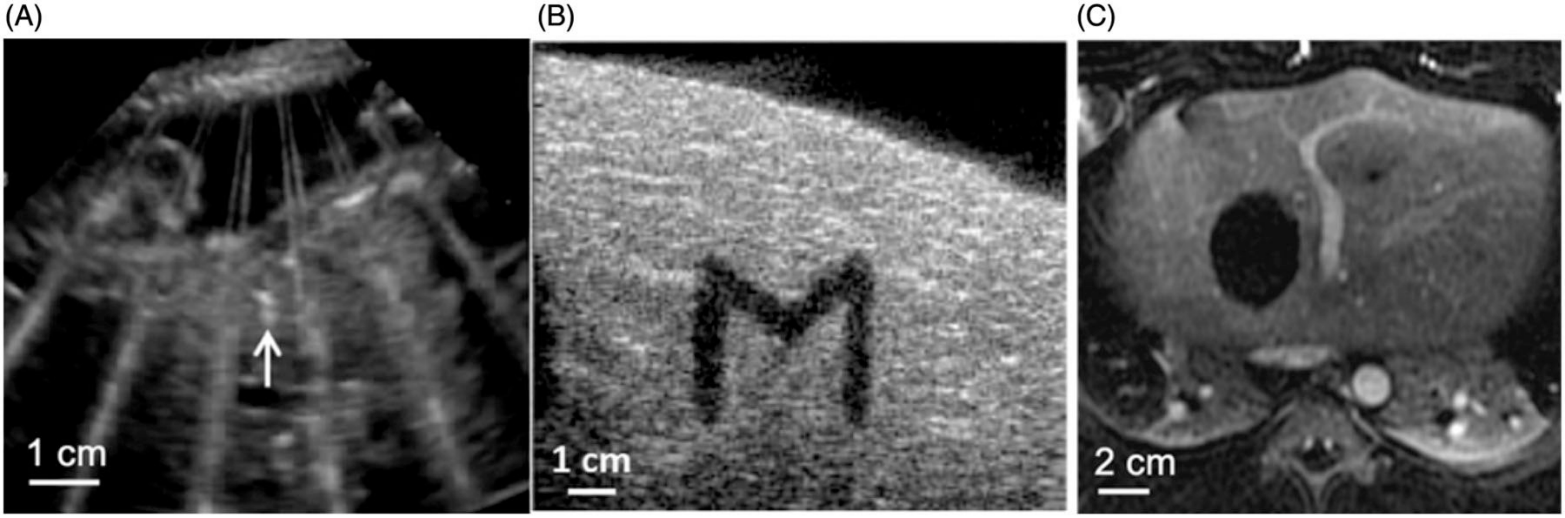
(A) Histotripsy-generated cavitation is seen as a hyperechoic zone on B-mode ultrasound. (B) ‘M’ shaped histotripsy lesion shows as a hypoechoic zone on B-mode ultrasound image. (C) Histotripsy ablation zone is seen as a hypointense (non-enhancing) region on this T1-weighted contrast-enhanced MR image.
